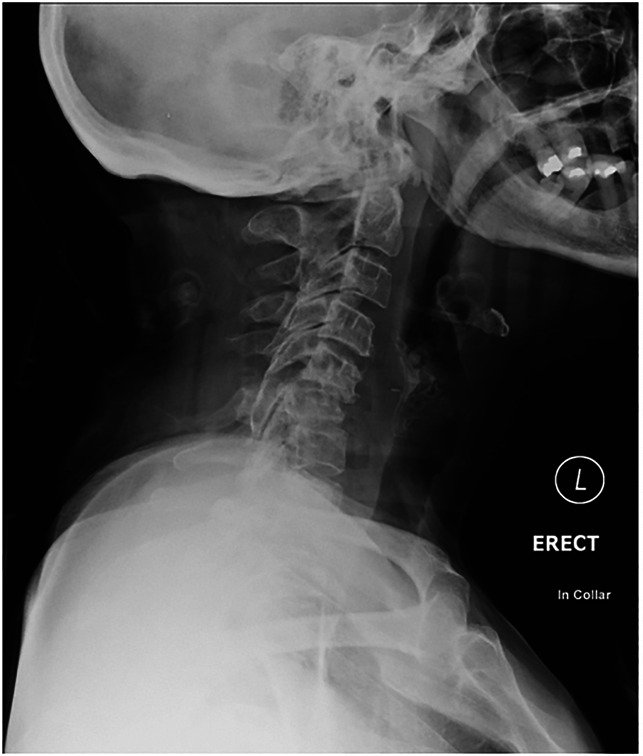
Exeter radiograph—erect lateral cervical spine radiograph demonstrating C5-C6 fracture subluxation.
